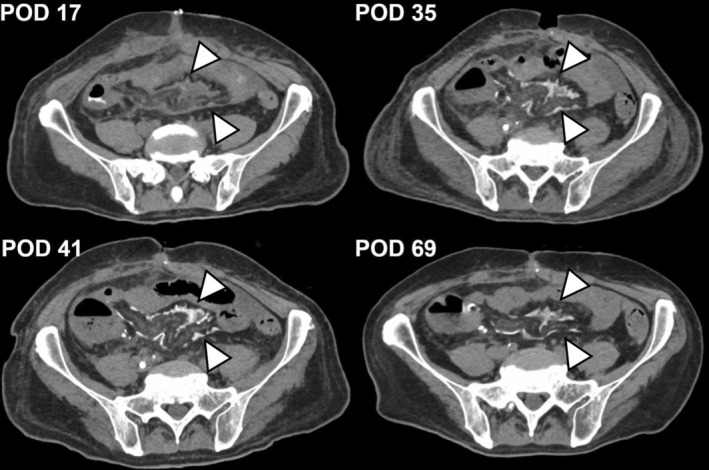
Sequential non‐contrast CT images. On POD 17, punctate high‐attenuation appeared within the contused mesentery (white arrows) after enteral diatrizoate administration. The attenuation intensified by POD 35 and 41, eventually fading by POD 69 as the patient's organ function improved. Note the circular high‐attenuation in the right common iliac vein on POD 35 and 41 represents an indwelling hemodiafiltration catheter.
